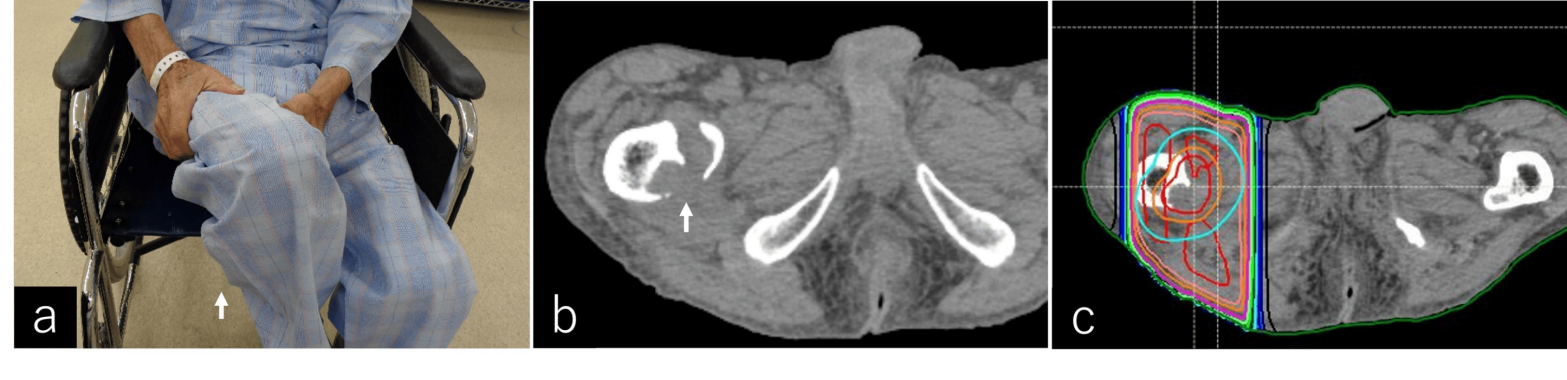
Case 1: Ishikawa-Teramura sign, CT imaging of metastatic mass, and radiation therapy planning. Ishikawa-Teramura Sign (IT sign) (a). A simple CT scan of the pelvic region revealed a metastatic mass and a pathological fracture at the right lesser trochanter of the femur (arrow) (b). Radiation therapy (RT) planning using RayStation (RaySearch Laboratories AB, Stockholm, Sweden) involved irradiation from two fields in the anterior-posterior direction. The patient received 25 Gy in 5 fractions of RT for the right femoral lesser trochanter (c).
